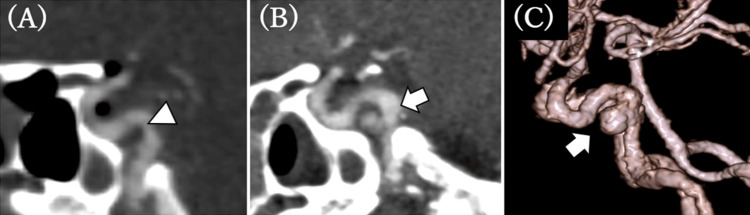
Comparison of CTA images from Day 0 (A) and Day 8 (B, C) (A) Initial CTA on Day 0 shows no evidence of an aneurysm (arrowhead). (B, C) Follow-up CTA on Day 8 demonstrates a newly developed aneurysm measuring 5 mm at its maximum diameter (arrow). CTA, CT angiography
